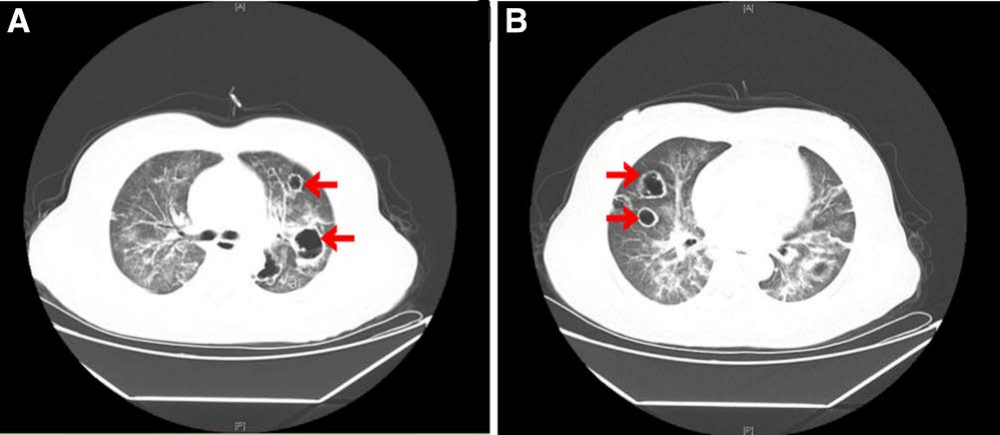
Computed tomography (CT) results of this patient. Chest CT of this patient showed multiple thick-walled cavities in both lungs (at the arrow mark), small amount of pericardial effusion; some mediastinal lymph nodes slightly enlarged, indicating a probability of inflammation of both lungs, not excluding mycobacterial infection.
